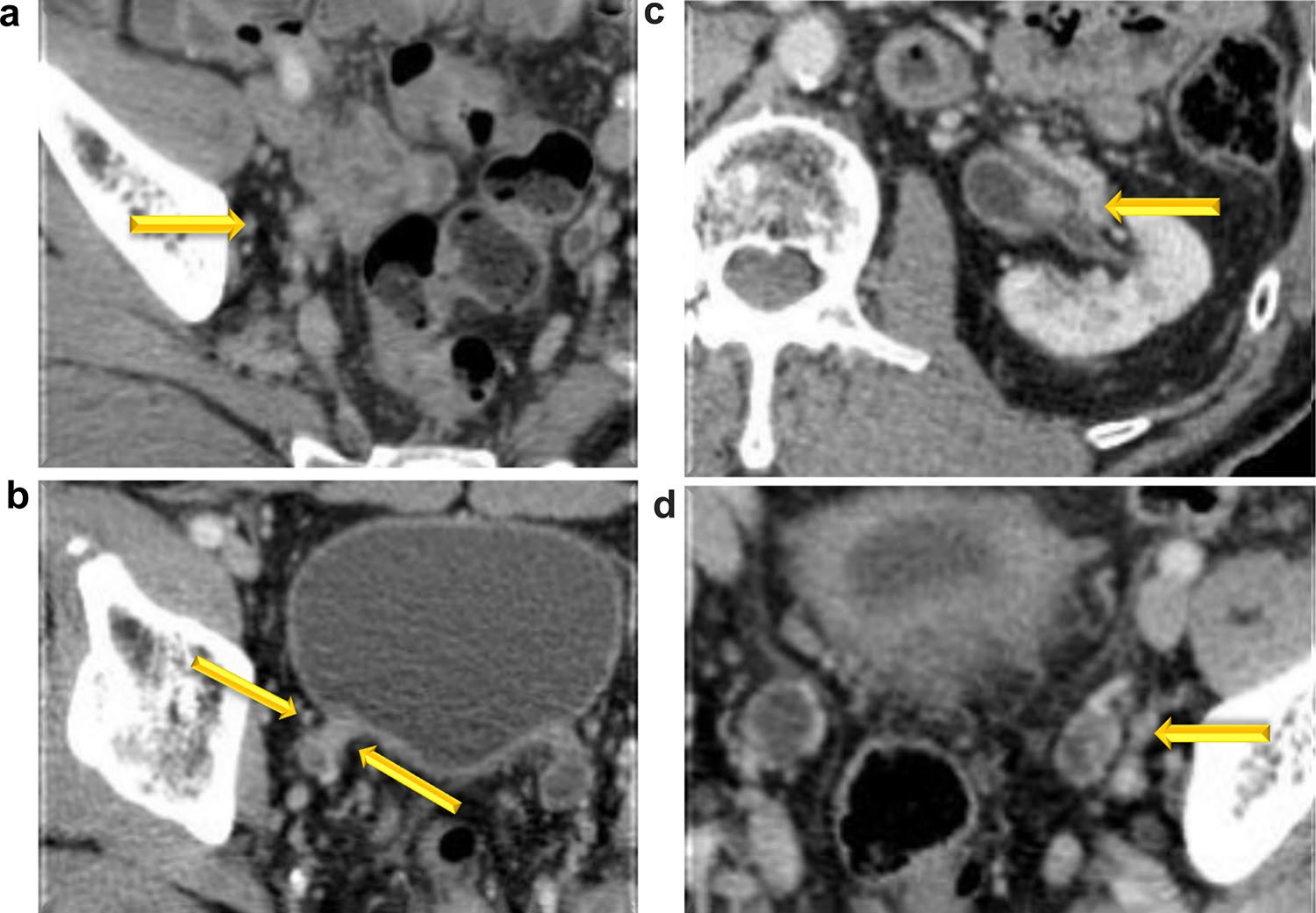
Pre-nephroureterectomy contrast computed tomography showed bilateral ureteral cancer (arrows). Right central ureter (a). Right lower ureter (b). Left renal pelvis (c). Left lower ureter (d)
